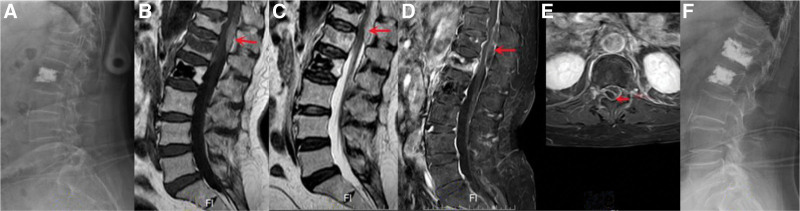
(A) X-rays showed vertebral compression fractures at T12 and L1, and a vertebroplasty of L2. (B, C) MRI showed acute compression fracture of L1 body and epidural hematoma extending from T12 to L1. (D, E) Enhanced MRI showed acute compression fracture of L1 body and epidural hematoma extending from T12 to L1 with annular enhancement. (F) X-rays showed that bone cement was well localized in the L1 vertebral body. MRI = magnetic resonance imaging.
